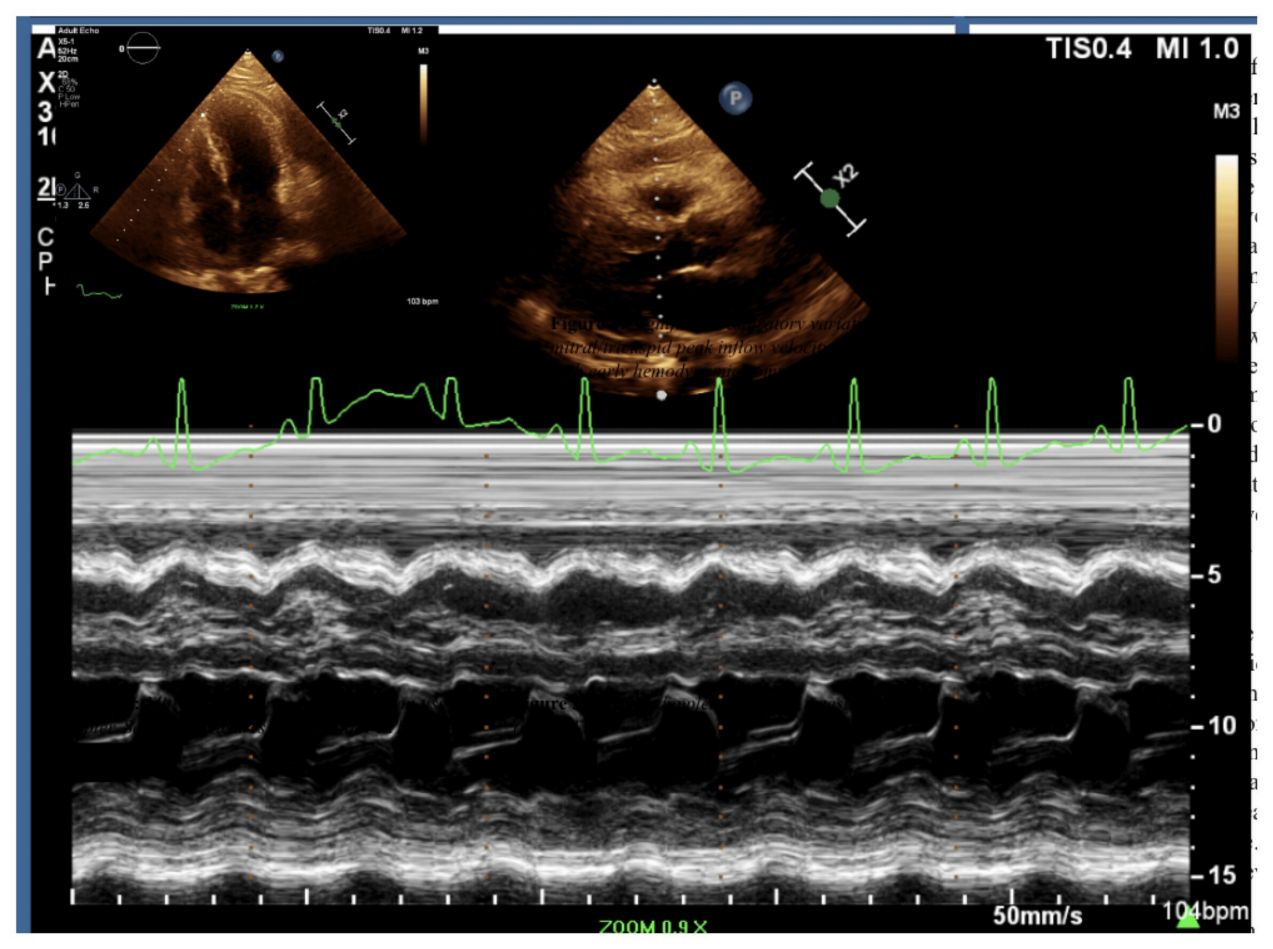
Pulsed wave Doppler assessment of mitral inflow velocities demonstrating marked respiratory variation (>25%) The finding is consistent with ventricular interdependence and early constrictive physiology on initial echocardiography
